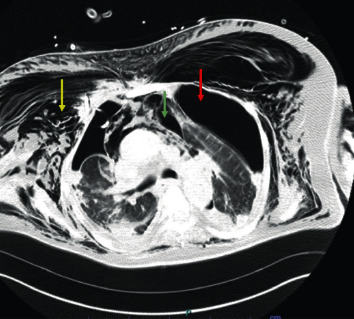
CT scan of the chest showing left-sided tension pneumothorax (red arrow), right-sided pneumothorax, pneumomediastinum (green arrow), and extensive subcutaneous emphysema of the chest wall (yellow arrow) with an indwelling right-sided chest tube.
